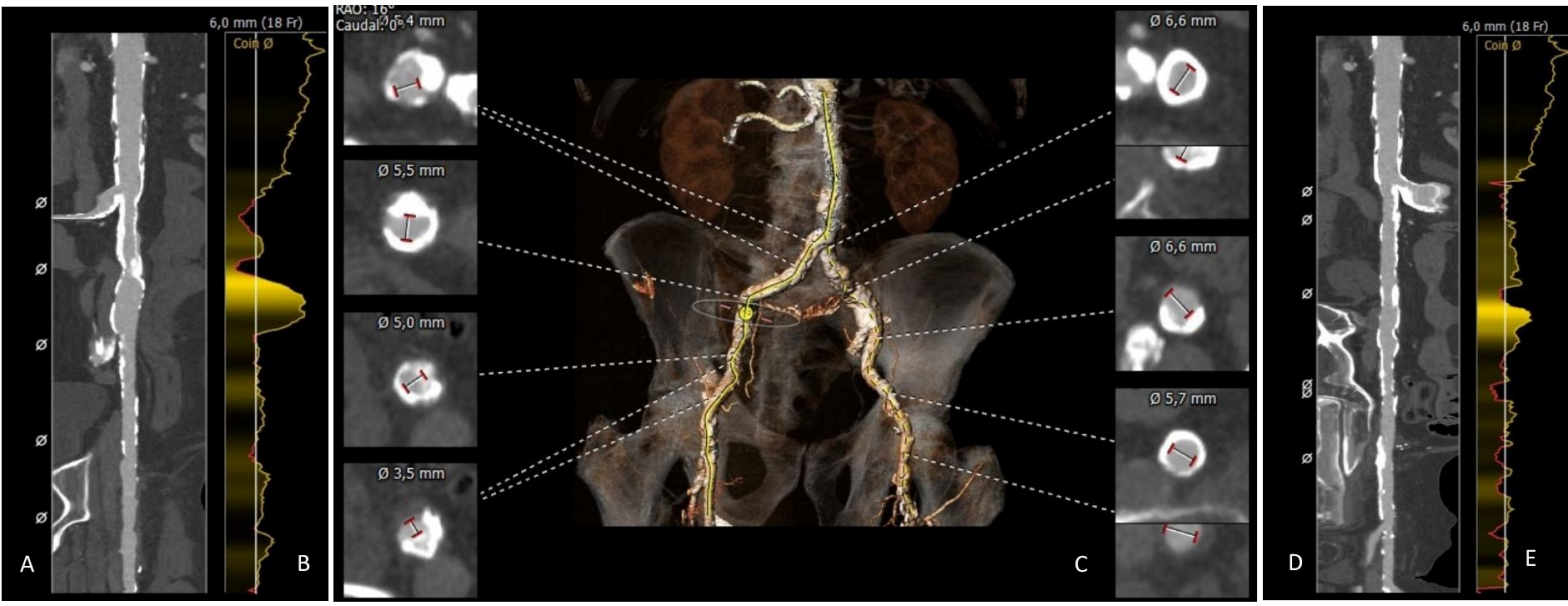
Computed tomography images of iliofemoral access. Stretched vessel views of the right and left iliofemoral artery (A,D) demonstrating advanced obstructive atherosclerotic disease with size of 6mm/18F minimal diameter bar set on the vessel diameter profile (B,E). En face view (C) of the iliofemoral arteries and aortic bifurcation and cross sections of most severe narrowing, with minimal lumen diameter of 3.6 and 2.9 in the right and left femoral artery, respectively.
